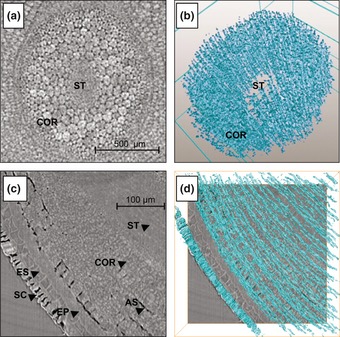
Three-dimensional model of void spaces in the hypocotyl of a developing oilseed rape (*Brassica napus*) seed. (a) CT image of the hypocotyl (corresponding to region ‘D’ in Fig. 1(c), cfr. horizontal section), where the stelar tissue is densely packed. (b) Model of void spaces in a segment of the hypocotyl. Channels in the cortex are aligned axially and are well inter-connected, while in the stele, pores are few in number. (c) CT image of the hypocotyl (corresponding to region ‘C’ in Fig. 1(c), cfr. vertical tangential section). (d) Model of void spaces in the same region described in (c). Here, there are a few longitudinal channels, but their inter-connectivity is poor. AS, airspace; COR, cortex of hypocotyl; ES, endosperm; EP, epidermis; ST, hypocotyl stele; SC, seed coat (testa).
